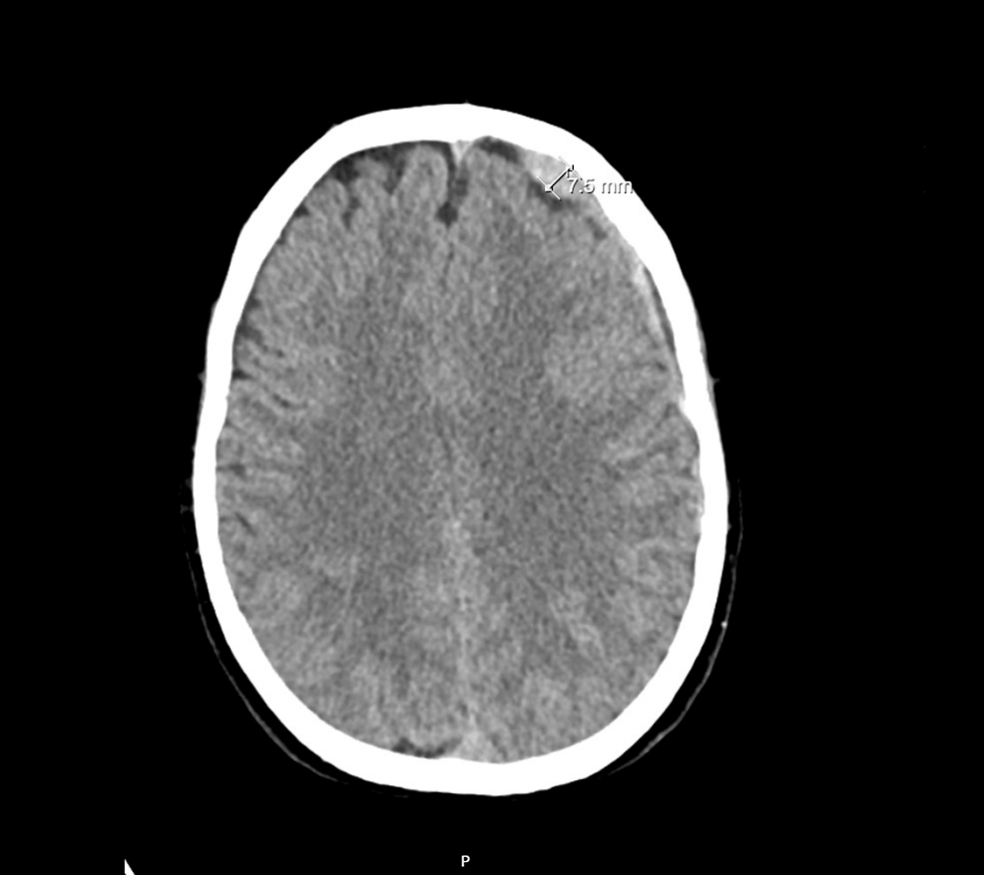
The patient’s cerebral CT scan revealed the presence of hygromas in the context of intracranial hypotension.
